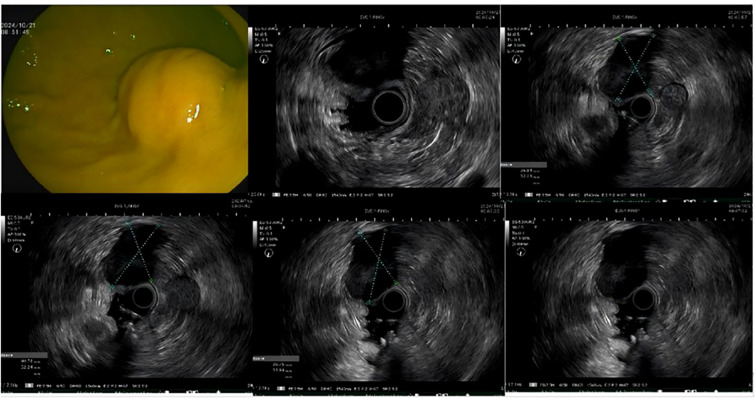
Ultrasound gastroscopy image.
